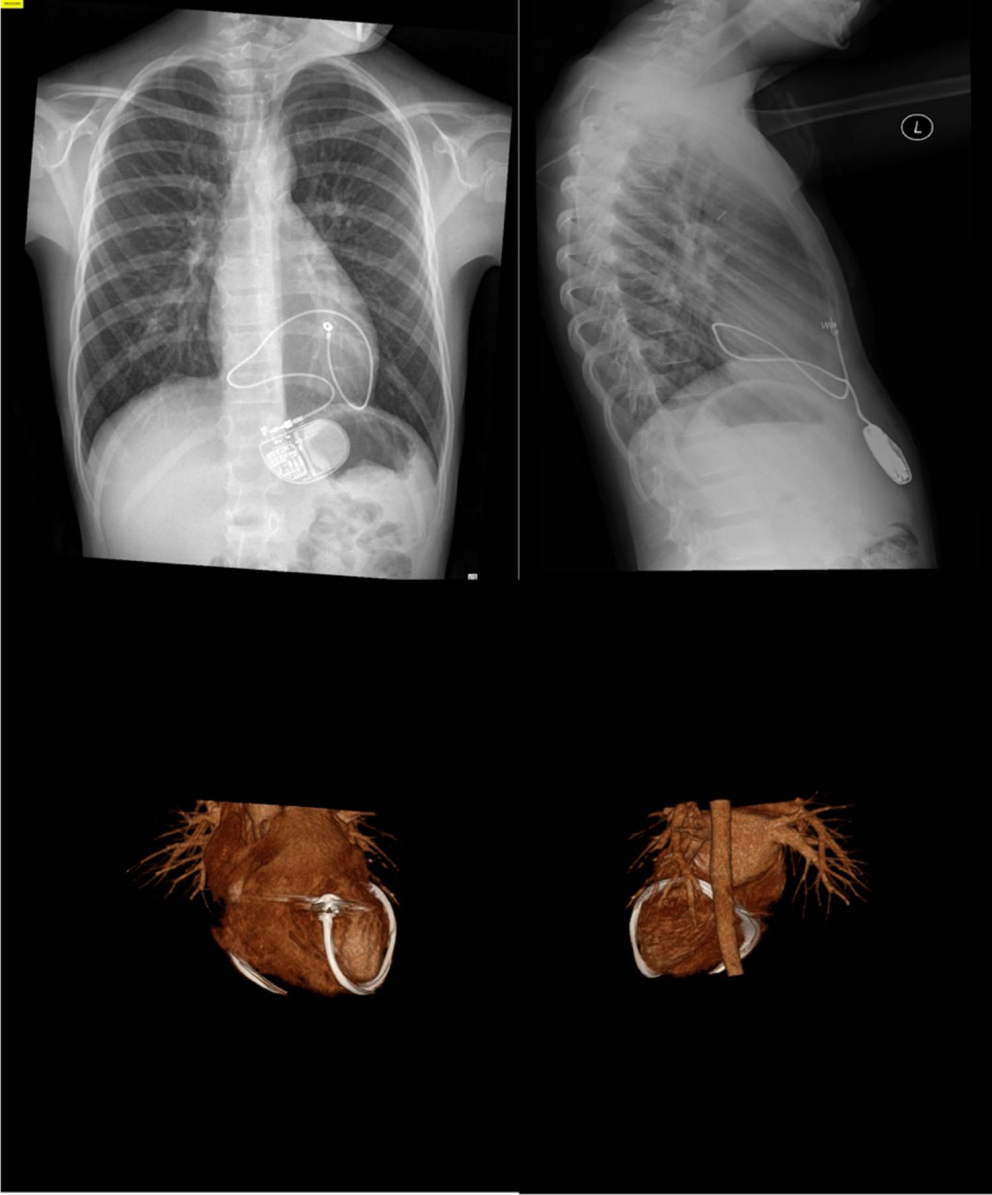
Appearance of a case initially mimicking strangulation with the appearance of plain radiography appearance (panel 1 &2), CT angiography confirming no evidence of indentation on the heart (panel 3&4)
